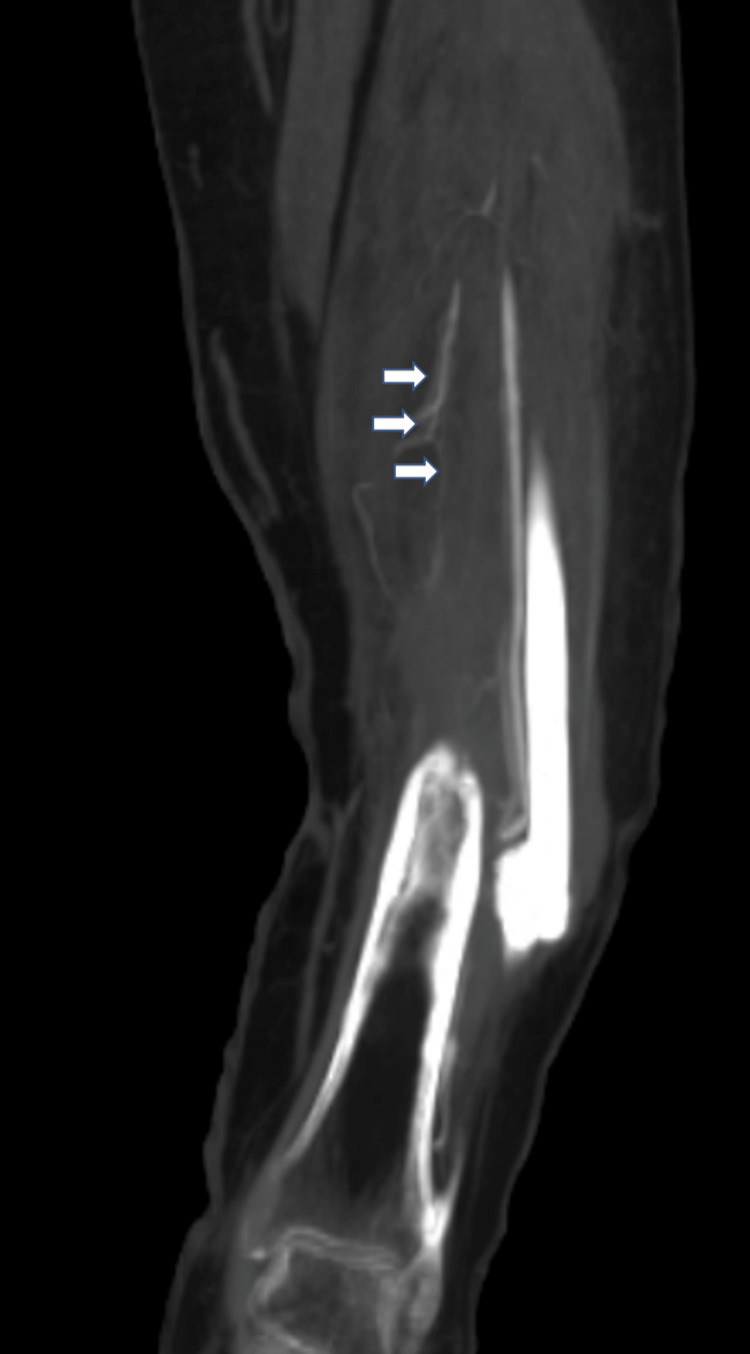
CT angiography revealing occlusion of left posterior tibial artery near the level of defect.
